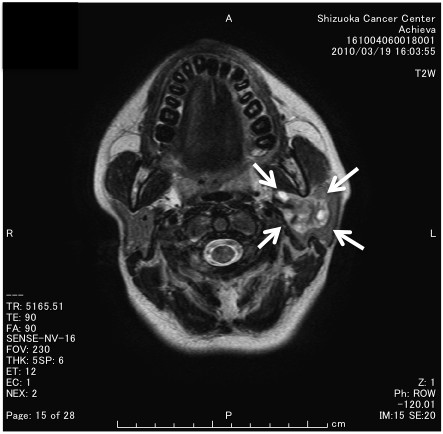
Magnetic resonance imaging (T2 weight imaging) showed an irregularly lobulated mass with irregularly low-intensity and partly high-intensity (white arrows) in the left parotid gland.
